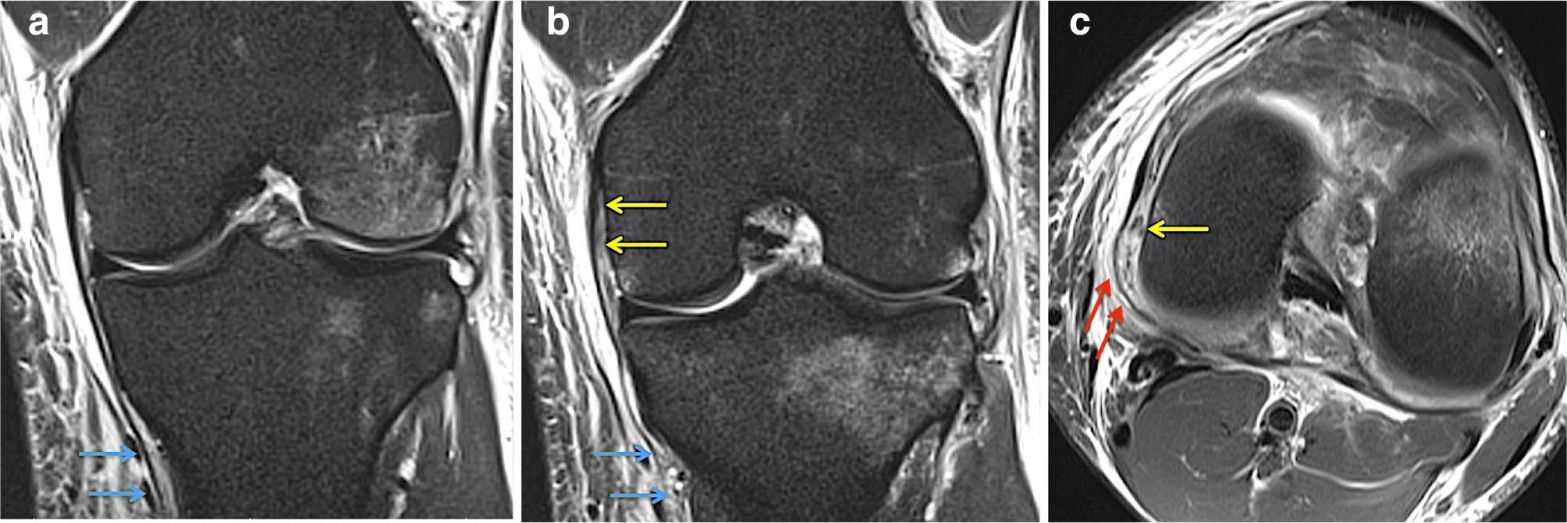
Sequential coronal (a, b) and axial (c) fat-suppressed proton density-weighted magnetic imaging shows a tibial superficial MCL lesion MRI grade II (blue), a deep MCL lesion at the meniscofemoral portion (yellow) and a disruption of the posteromedial capsule and POL (red) with surrounding soft tissue oedema
